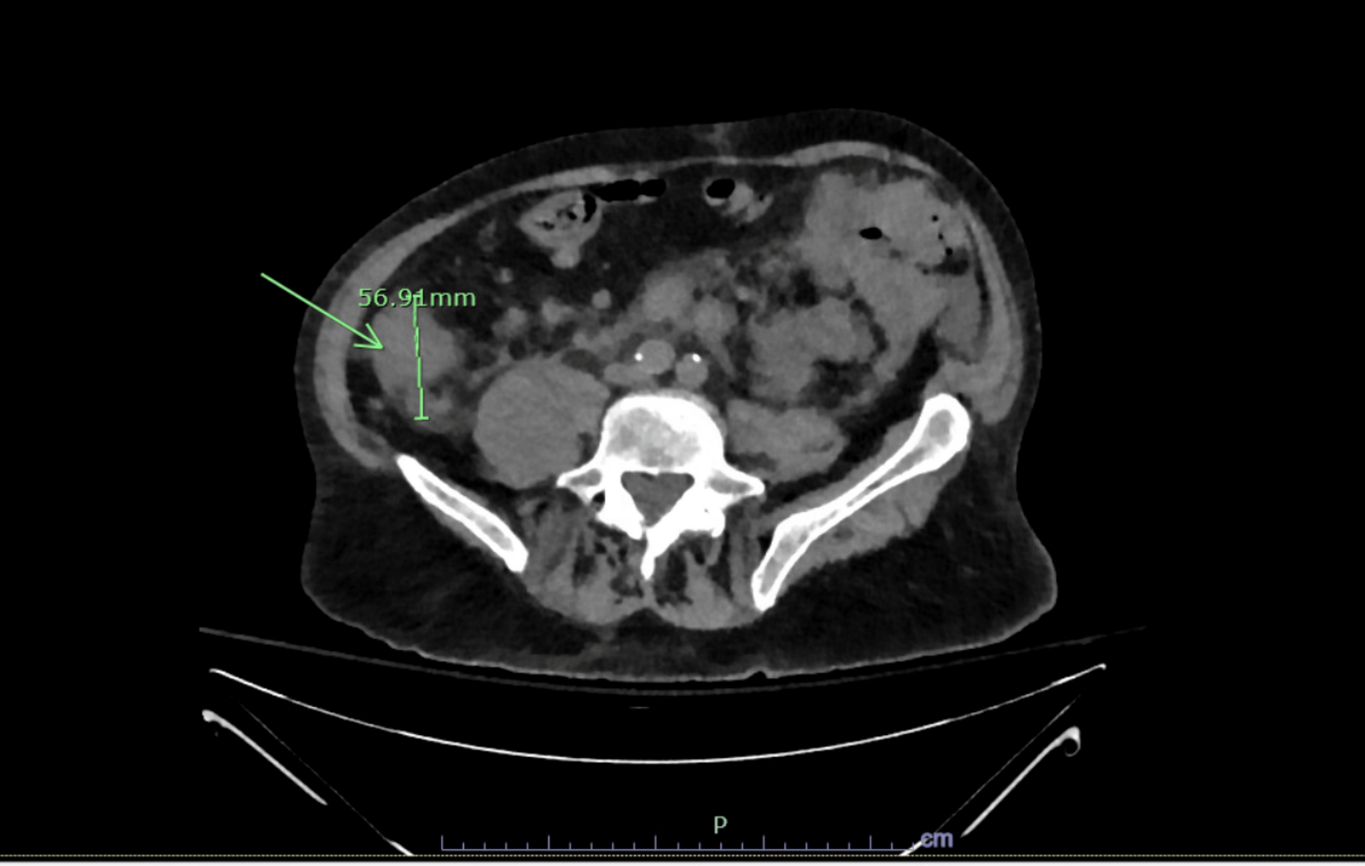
CTAP demonstrating a 5.7 cm retroperitoneal soft tissue implant CTAP, computed tomography of the abdomen and pelvis
